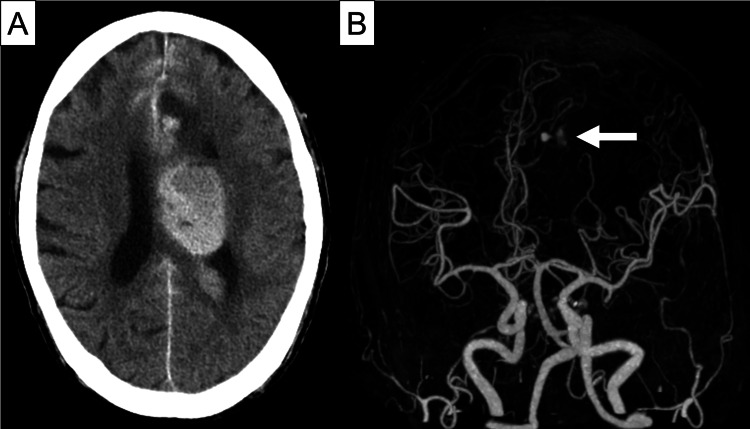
Head computed tomography (CT) and CT angiography (CTA) on day 52. (A) Head CT showing subarachnoid and intraventricular hemorrhage, necessitating immediate external ventricular drainage to manage intracranial pressure. (B) Head CTA revealing an aneurysm with extravasation of the peripheral segment of the left anterior cerebral artery (arrow), indicating the urgent need for intervention to treat the aneurysm and prevent further bleeding.
